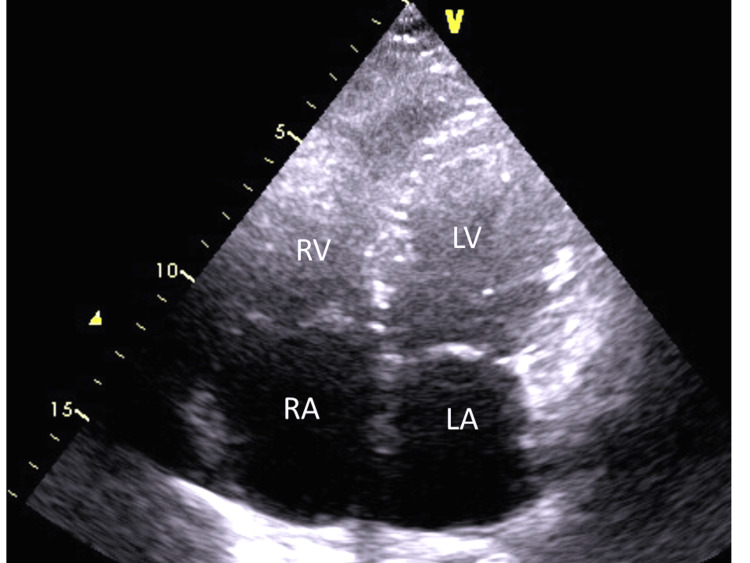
Echocardiogram eight years after the intervention. Apical four-chamber view. RV, right ventricle; RA, right atrium; LV, left ventricle; LA, left atrium
